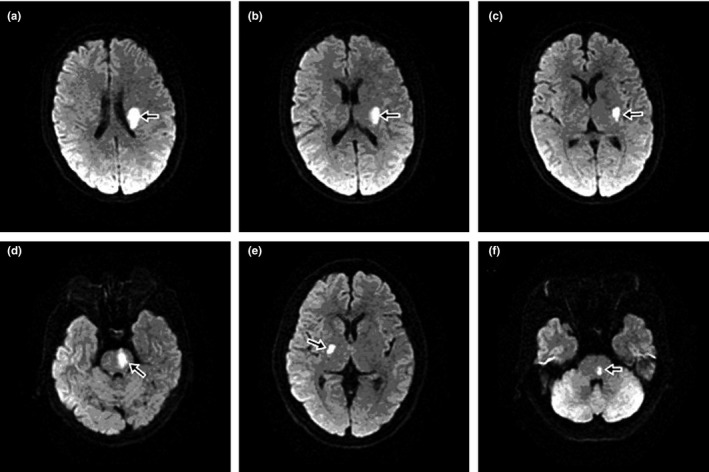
DWI findings in axial section (infarcts indicated by black arrow). (a, b, c) BAD in LSA territory. (d) BAD in PPA territory. (e) LD in LSA territory. (f) LD in PPA territory
